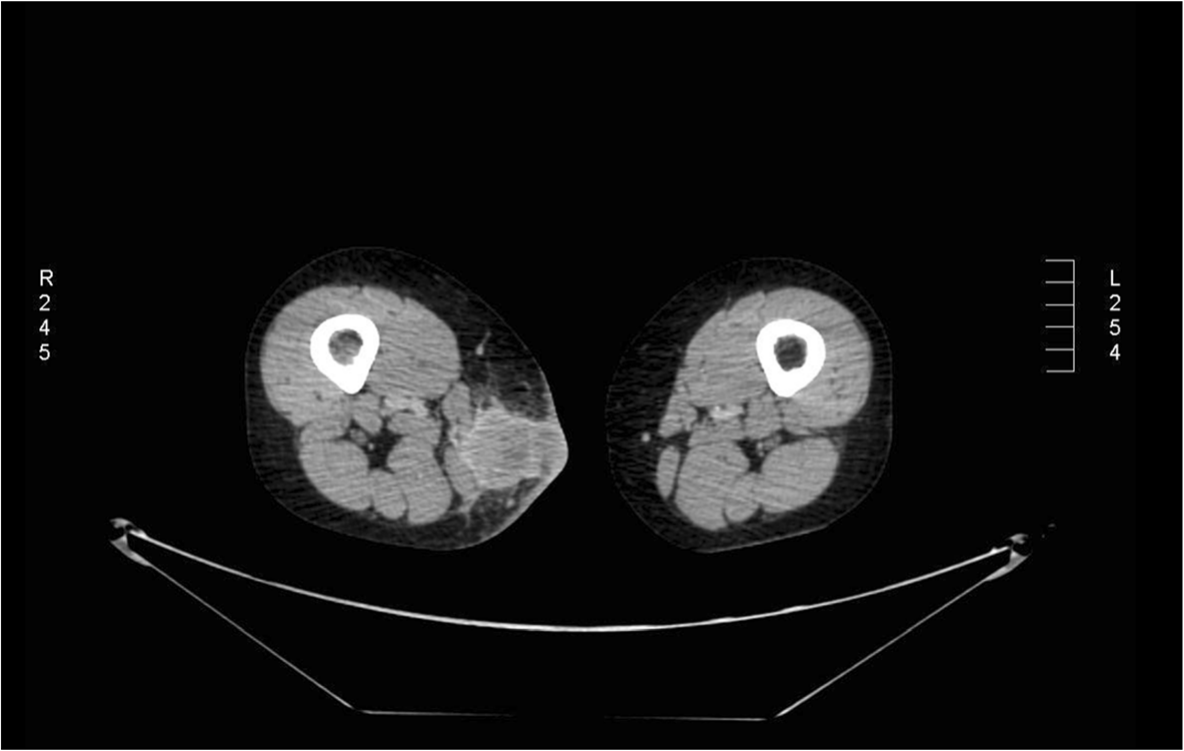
An axial section of a computed tomography scan showing a lesion in the soft tissue of the medial compartment of the right thigh.
